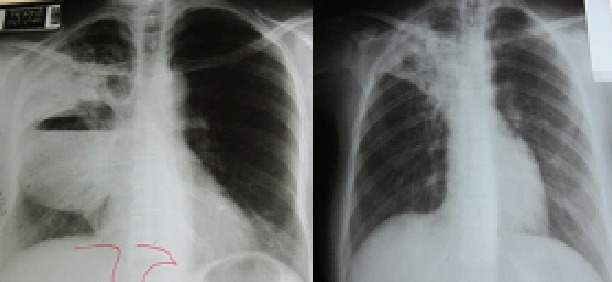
Radiographies thoraciques montrant des PT excavées du poumon droit
